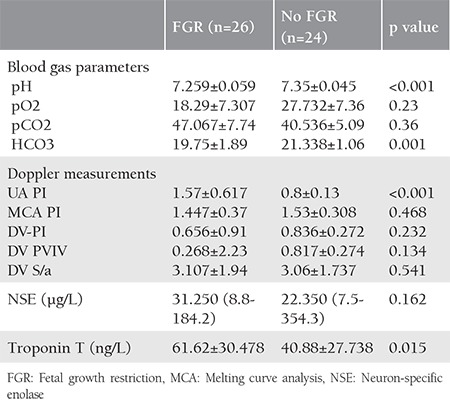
Blood gas analyses and Doppler velocity measurements in groups with and without FGR
